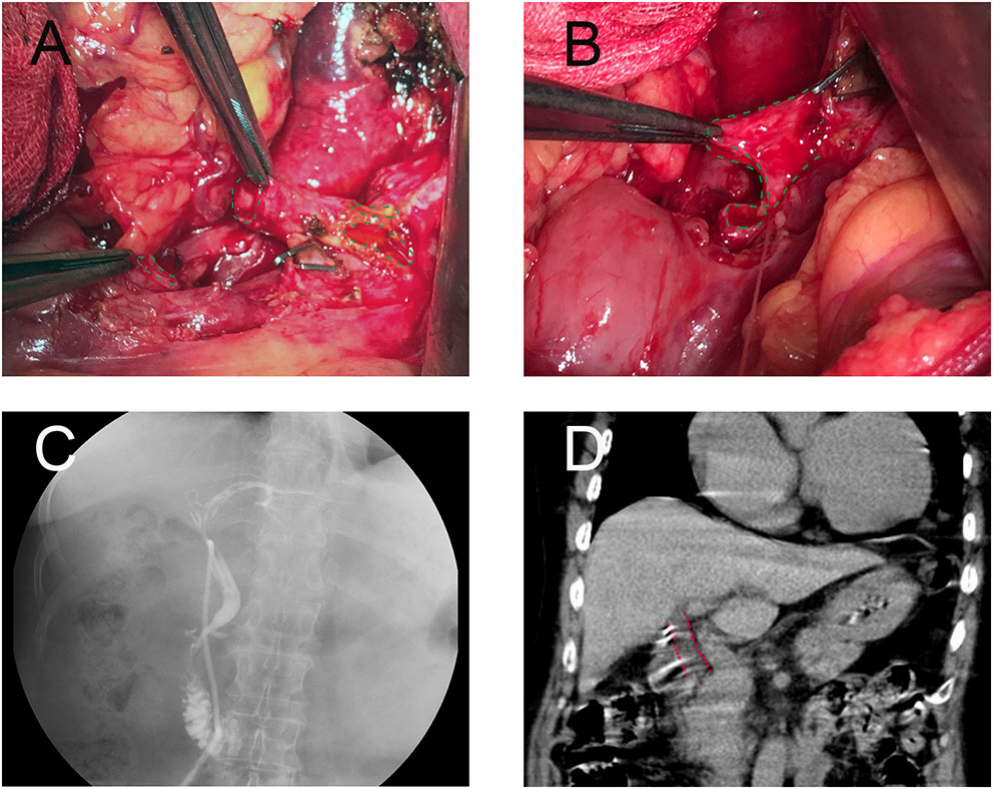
A patient with type E2 combined with type D (Strasberg-Bismuth) bile duct injury (BDI) underwent FMS end-to-end biliary-to-biliary reconstruction. (A) Intraoperative photograph of bile duct before shaping, bile duct gaps were represented by a green dashed line. (B) Intraoperative photograph of bile duct after fish mouth shaping, the bile duct was reshaped as a fish-mouth. (C) T-tube cholangiography before T-tube removed. (D) CT-scan at year 2 postoperative. The common bile duct was highlighted (red dashed line), no stenosis was found.
